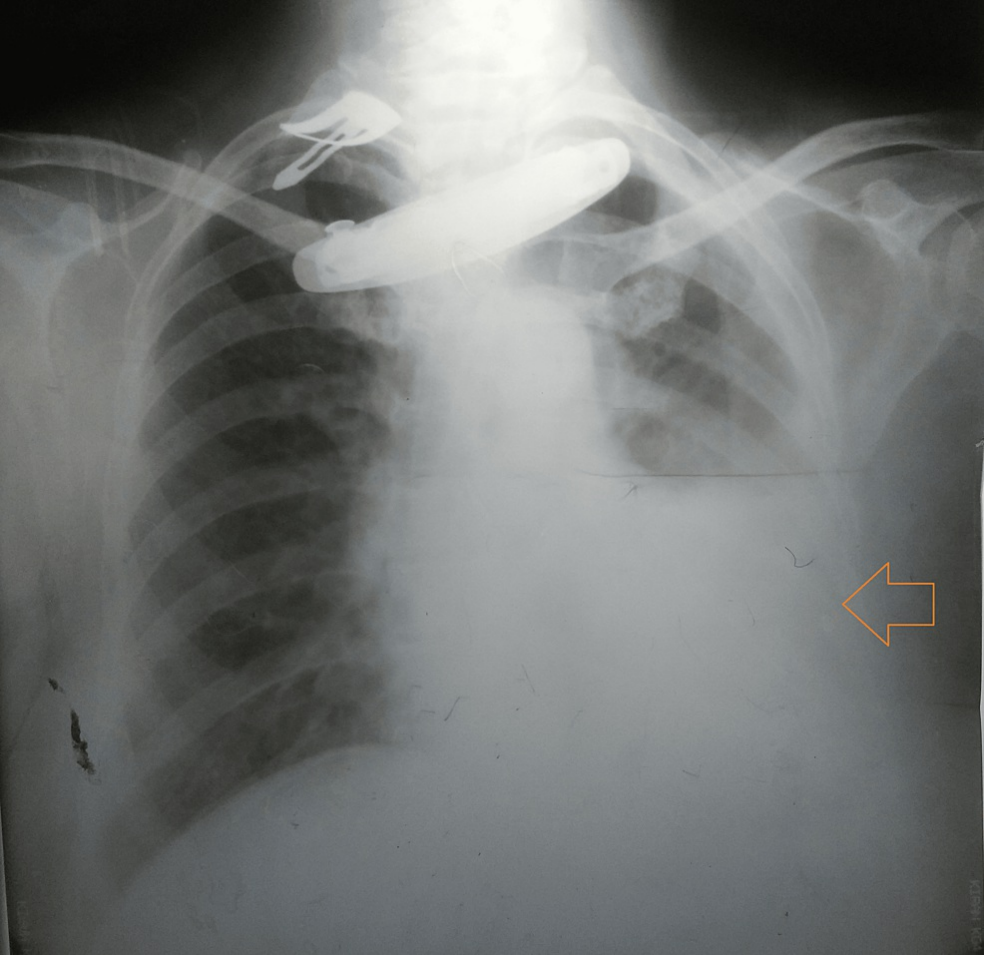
Chest radiograph (PA view) suggesting cardiomegaly with left pleural effusion PA: Posteroanterior
